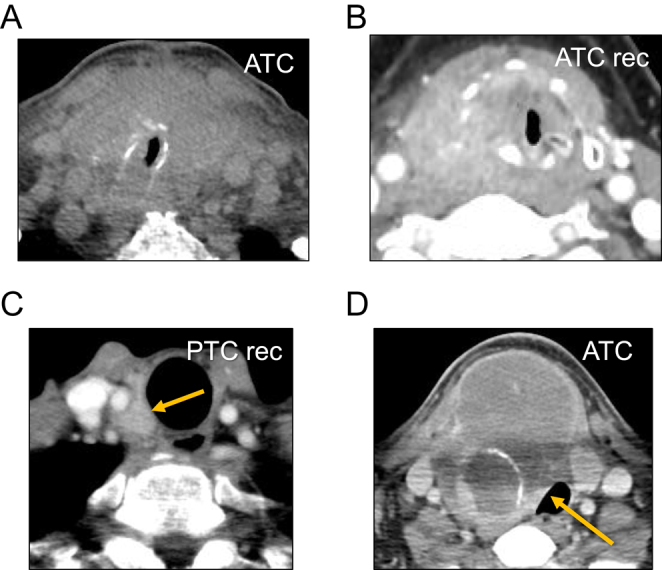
Typical CT scans images of airway involvement. (A and B) Remarkable invasion into trachea (A) or larynx (B) with remarkable shift and stenosis. (C and D) Typical cases of tumors that were widely attached to trachea without infiltration into the lumen, with preserved border of the tracheal wall. These cases were excluded from the present study, suspicious of tracheal involvement; rec, recurrence.
